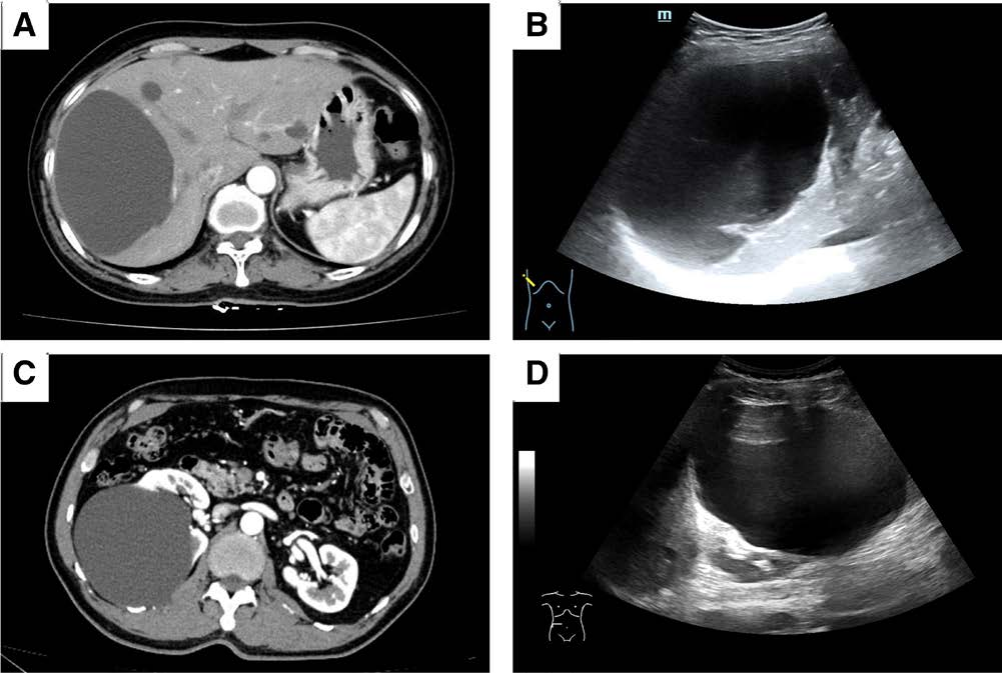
Preoperative CECT and US to evaluate the size and location of hepatic and renal cyst. (A) Characterization of giant hepatic cysts in CECT. (B) Ultrasonographic image of a giant hepatic cyst. (C) Characterization of giant renal cyst in preoperative CECT and exclusion of other lesions. (D) Giant renal cyst in US sonography. CECT = contrast-enhanced computed tomography, US = ultrasound.
